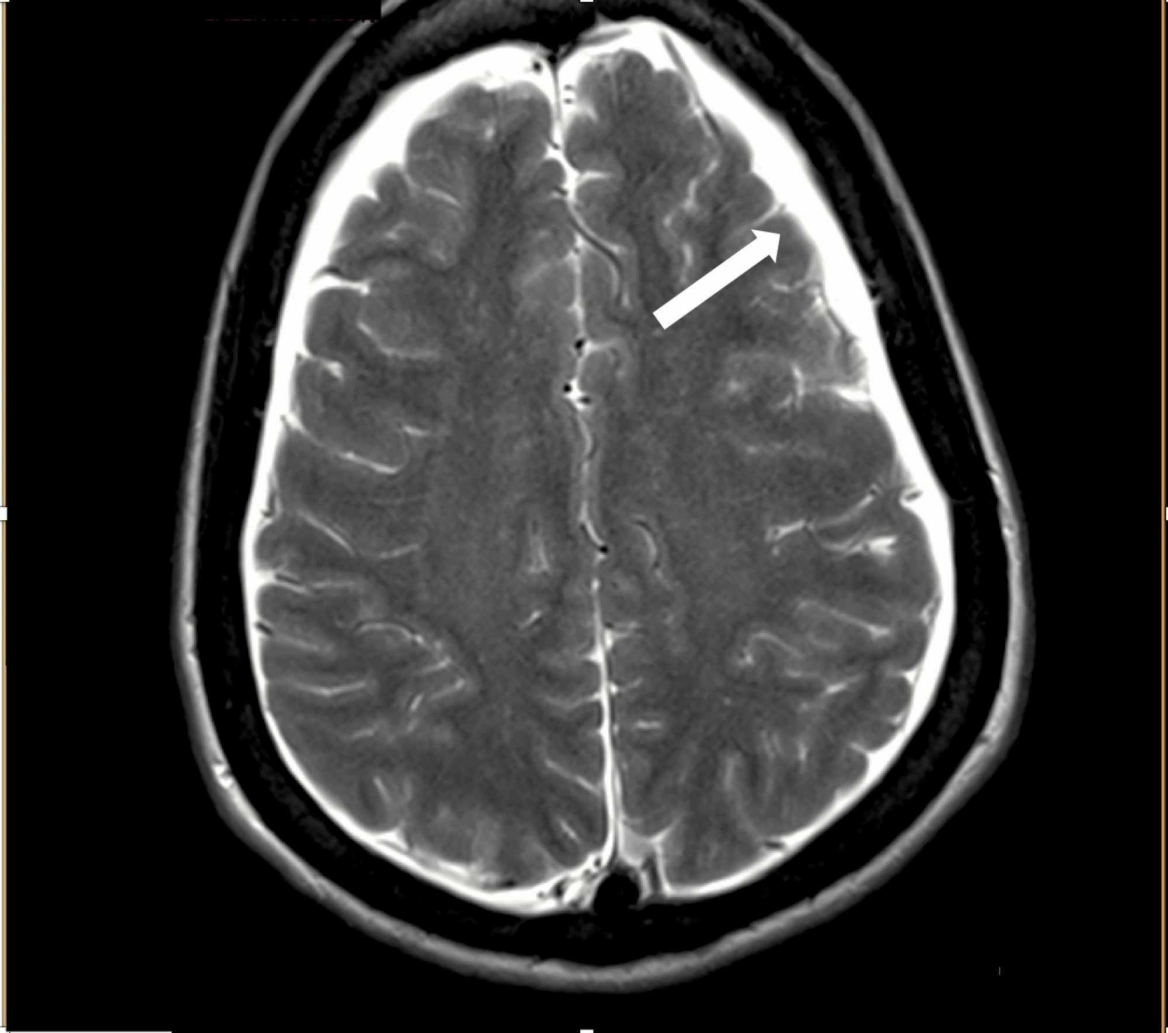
Coronal view magnetic resonance imaging (MRI) depicting subdural effusions (white arrow)
